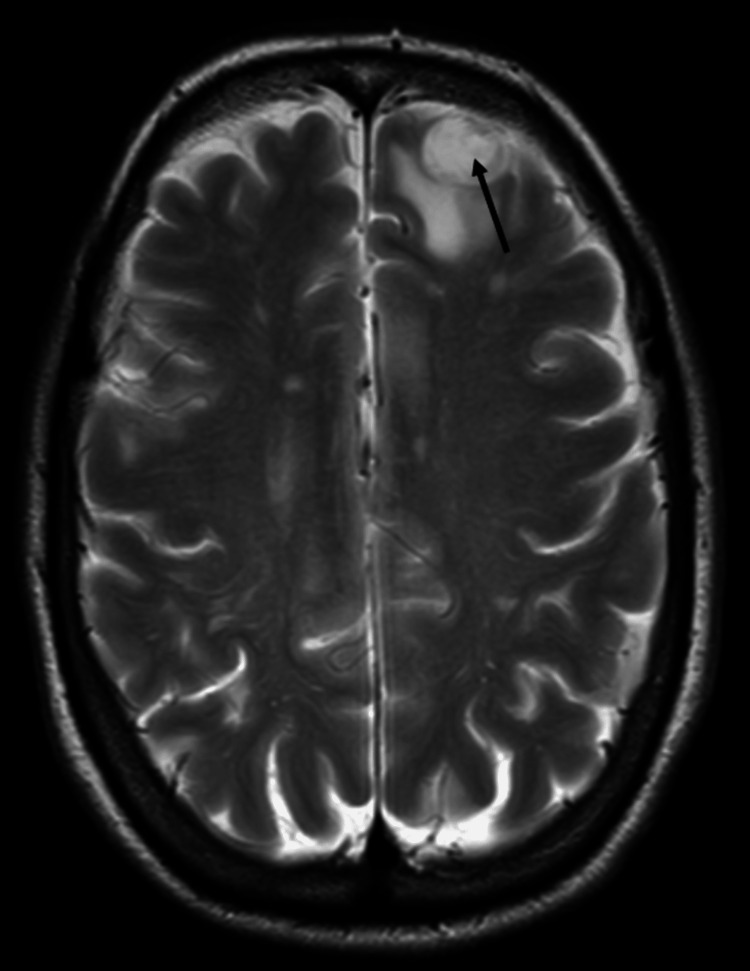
MRI of the brain showing the single 15x13 mm metastasis (arrow) with surrounding vasogenic edema in the left anterior frontal lobe
